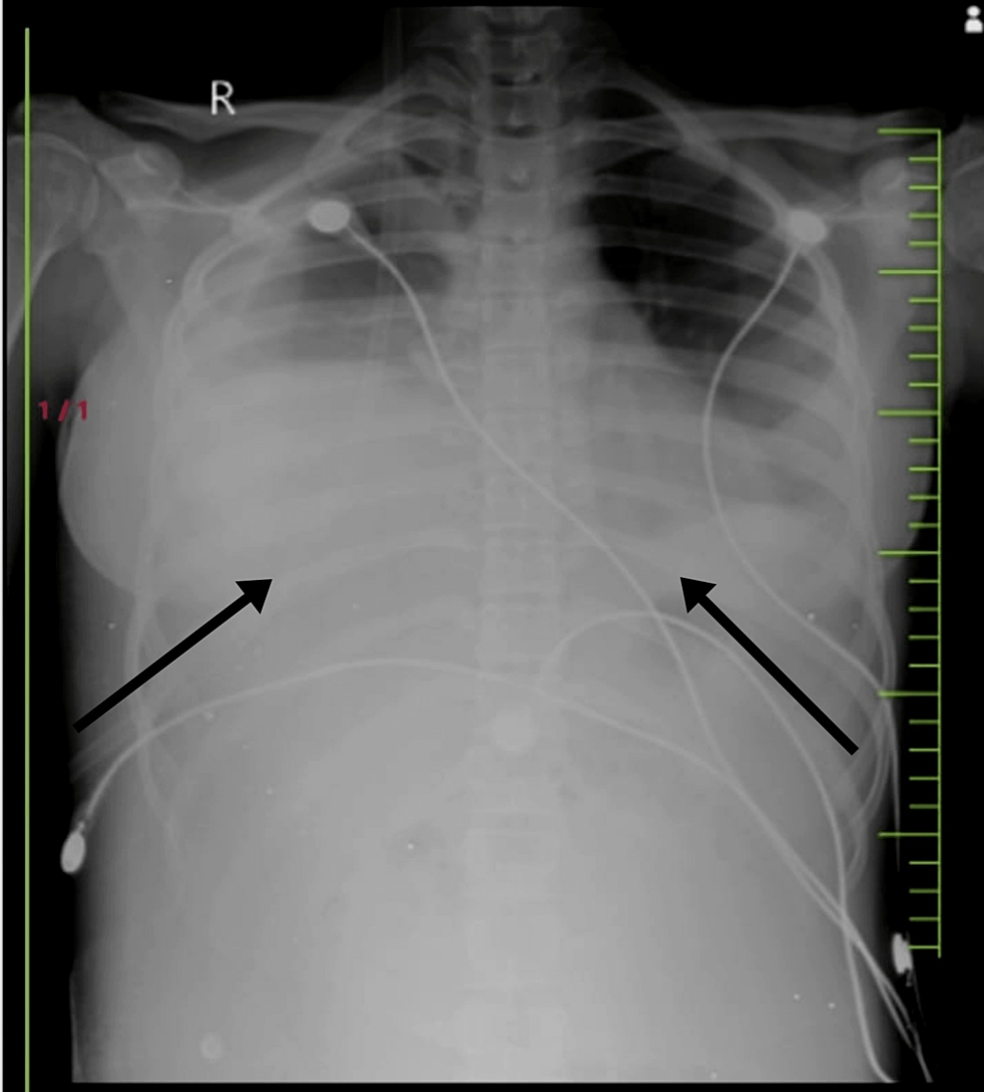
Chest X-ray on day 4. The chest X-ray shows significant obliteration of the bilateral costophrenic angles. It indicates moderate pleural effusion. The X-ray was advised when the patient suddenly desaturated to 89% on room air and started complaining of breathlessness and dyspnoea.
